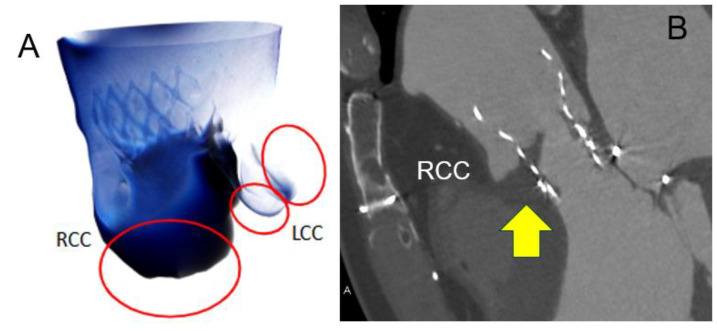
Compute tomography blood flow analysis around sinus of Valsalva in a case after transcatheter aortic valve replacement. (A): The computed tomography (CT) flow analysis of this case after transcatheter aortic valve replacement shows a marked decrease in blood flow (dark blue lesion) in the right coronary cusp (RCC) of sinus of Valsalva (SOV) (red circles mean each cusp of SOV, LCC means left coronary cusp). (B): A huge thrombus was detected in the RCC of SOV on CT in the same patient (yellow arrow).
